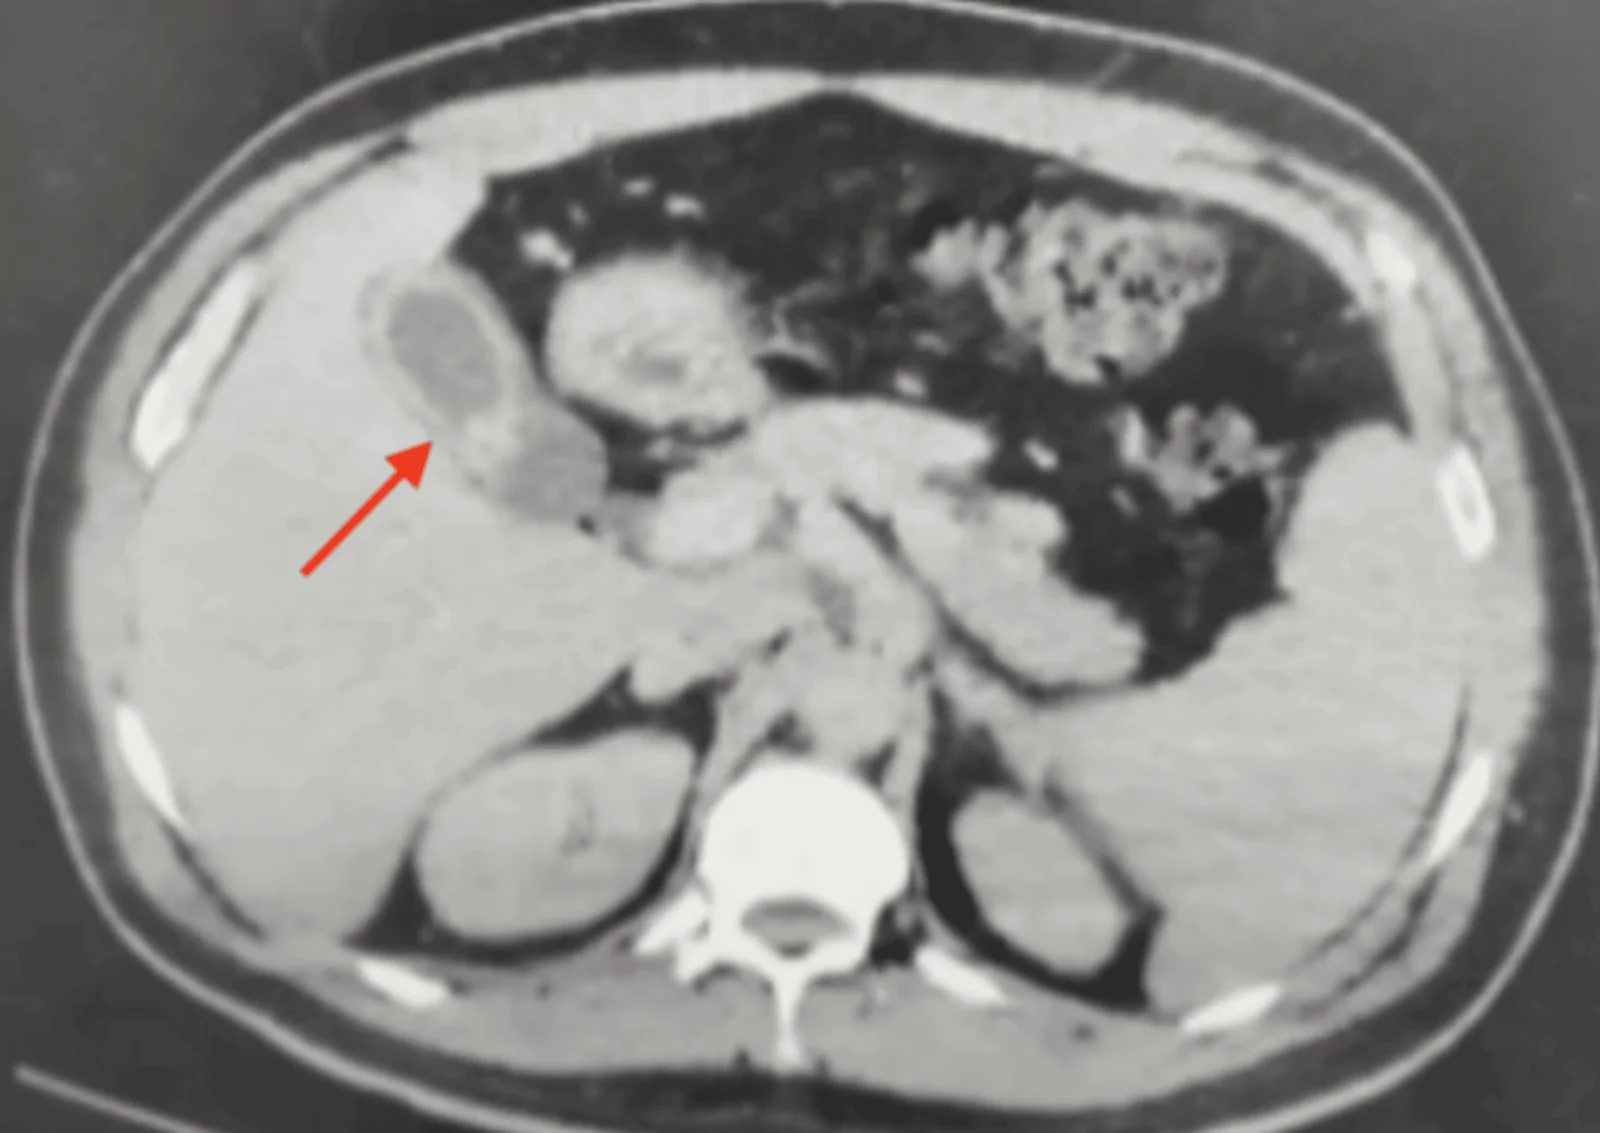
Gallbladder on CT scan, axial view. The red arrow indicates a thickened gallbladder wall with pericholecystic fluid and a stone.
